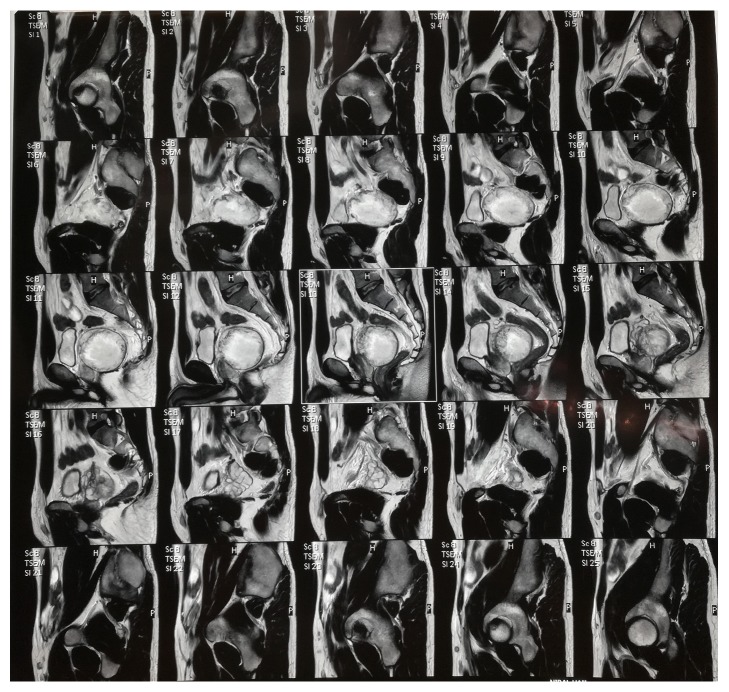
Pelvic MRI showing a large mass confined to the pelvis measuring 7.2 cm in the largest diameter with predominance of cystic component without evidence of fatty content, calcification, fluid-fluid level, or suspicious enhancing nodular soft tissue thickening.
